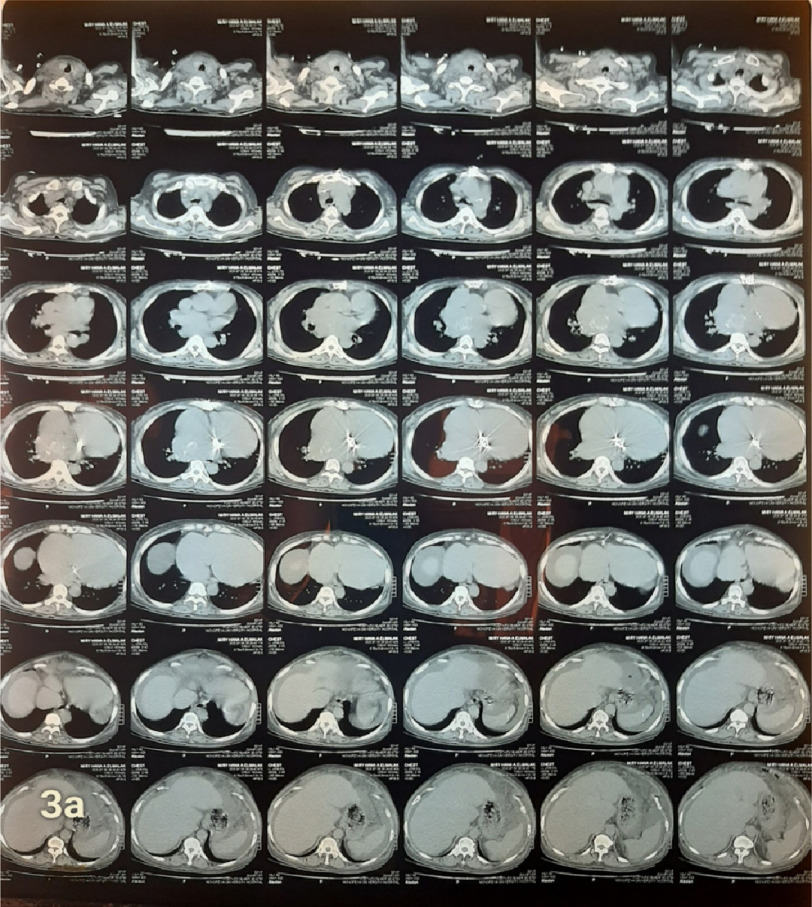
Computed tomography of the patient showing dilated cardiac chambers and round mass in the LA.
